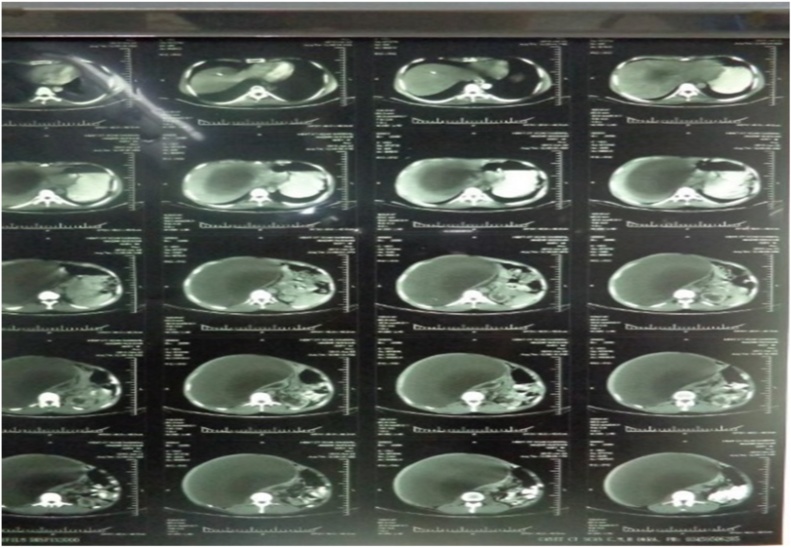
CT scan showing retroperitoneal Cyst displacing right kidney (case 2).
